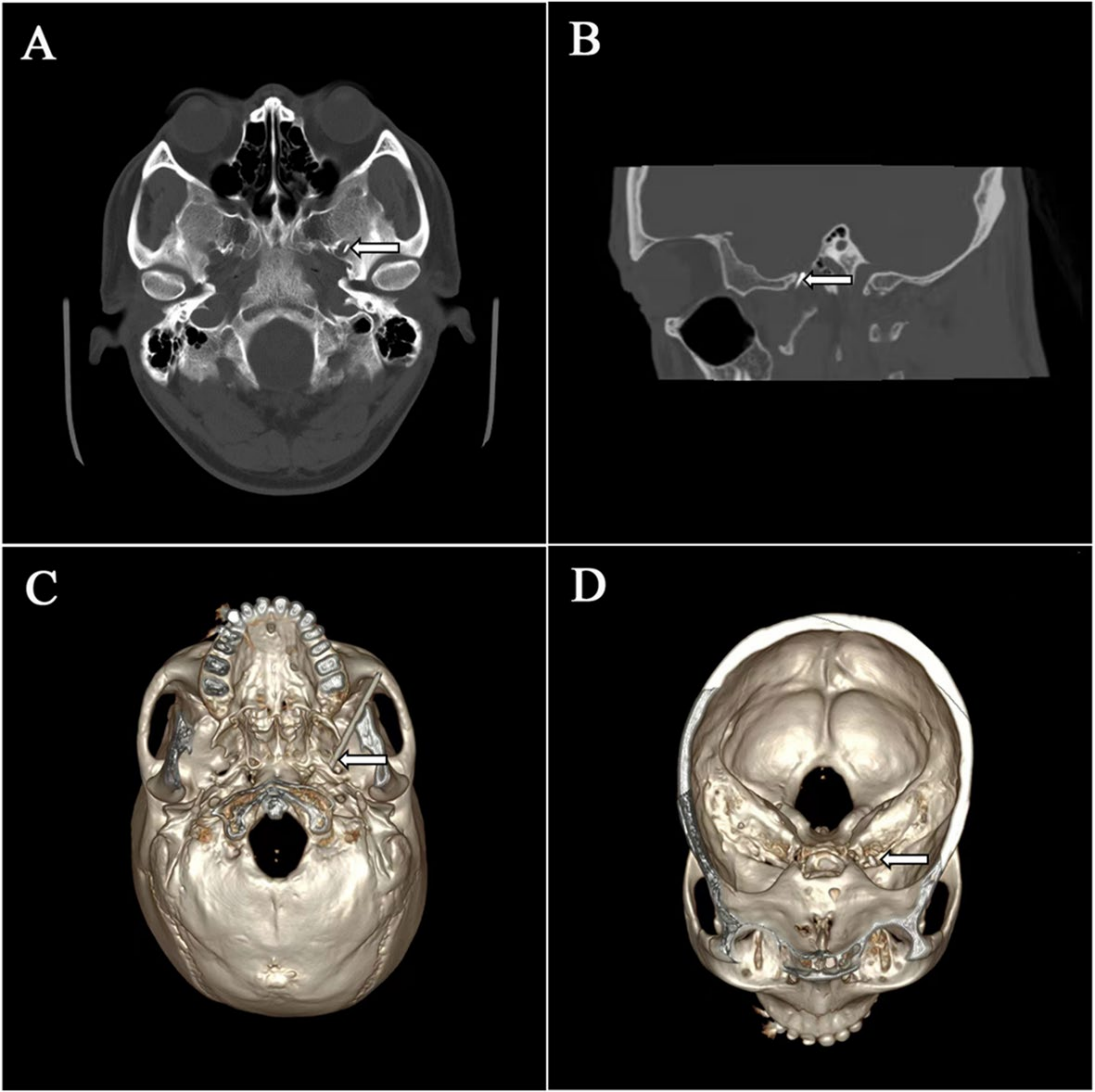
CT-guided puncture of the Gasserian ganglion. A Axial CT scan showing the needle (arrow) entering the foramen ovale. B Sagittal CT scan showing the needle (arrow) entering the foramen ovale. C 3D CT reconstruction of skull base view showing that the trocar entering the foramen ovale. D 3D CT reconstruction of interior skull base view showing that the trocar entering the foramen ovale
